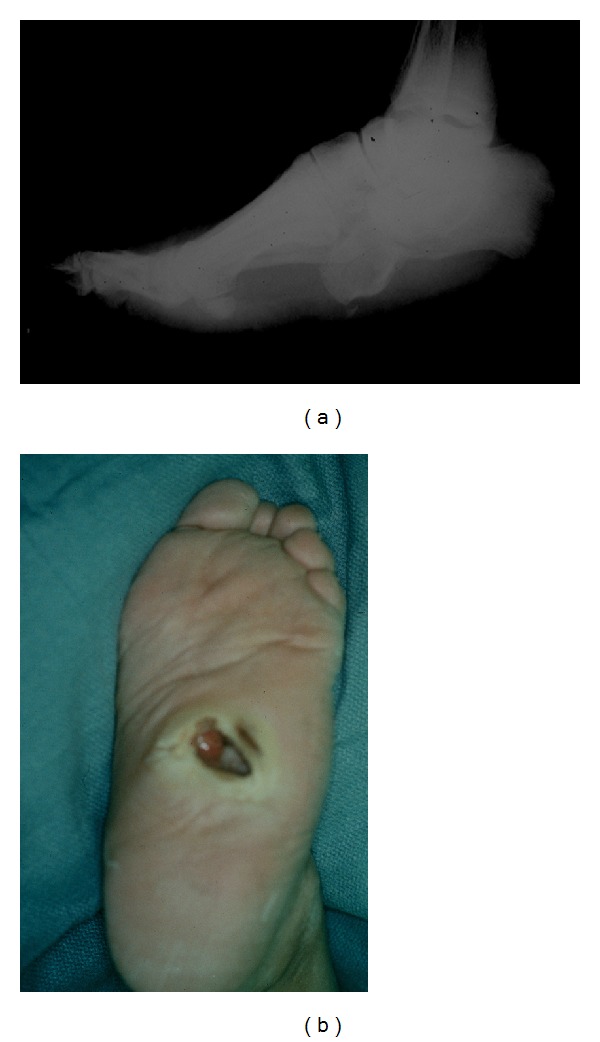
Rocker bottom foot deformity secondary to Charcot disease. (a) X-ray image lack of ankle joint dorsiflexion resulting in an increased load to the forefoot. This in turn leads to collapse of the tarsometatarsal joint, which is the focus point between the forefoot and hindfoot. The arch has collapsed and appears to be almost inverted. (b) Charcot foot with rocker bottom has increased propensity to pressure ulcers formation and is often seen with the involvement of Lisfranc joint.
